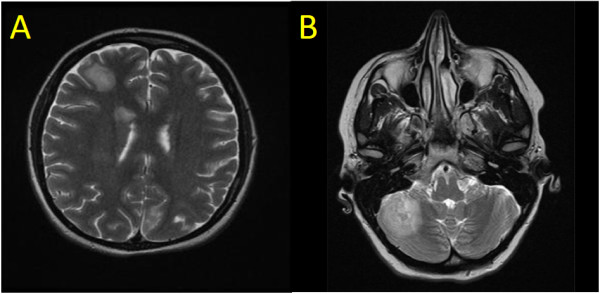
Central nervous system lesion in our case. T2 weighted MR image showing a large hyperintense lesion in A) right frontal cerebral cortex and B) right cerebellar cortex.
